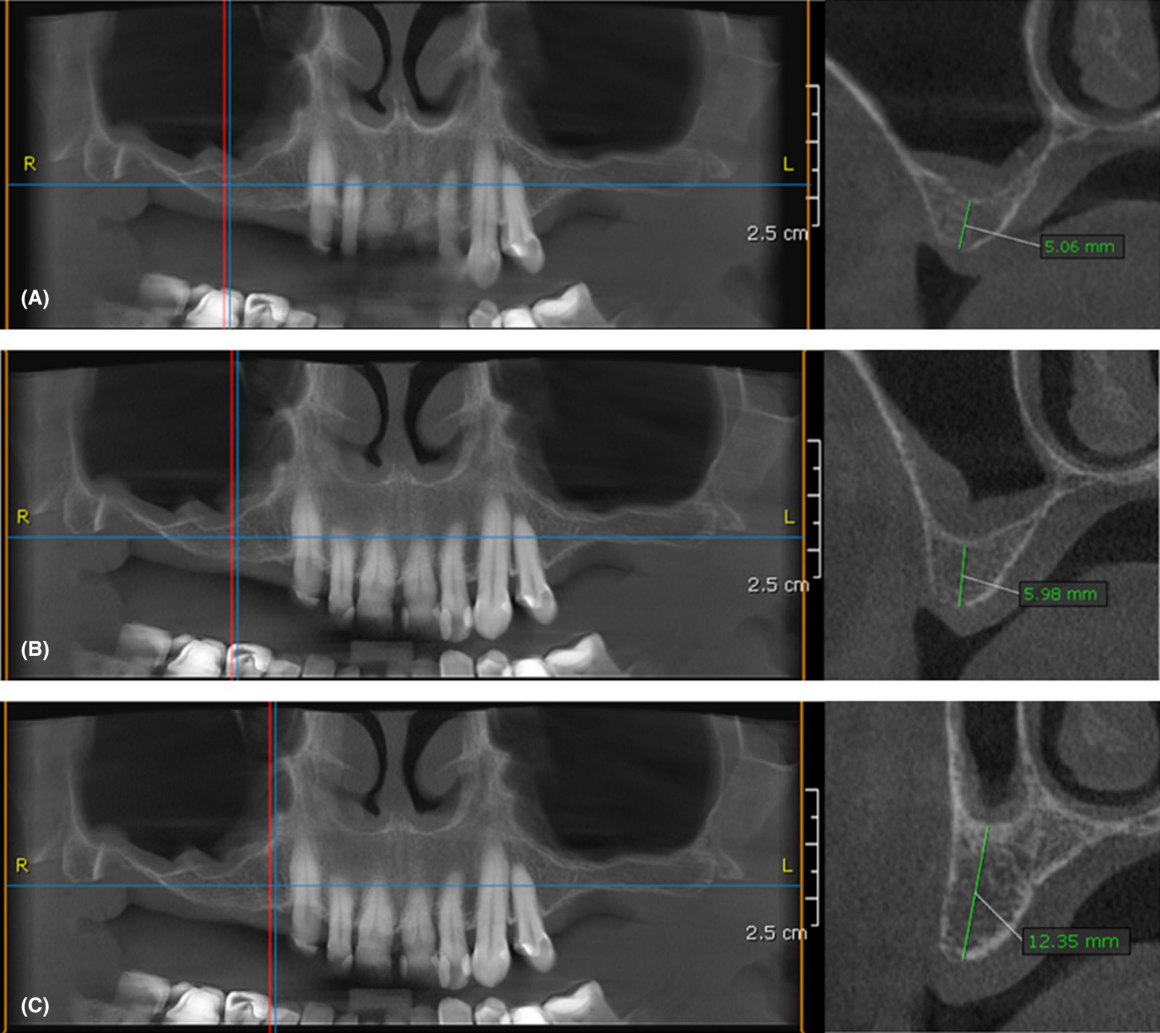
CBCT scan of the maxilla before implant placement. (A) Bone dimensions at the edentulous site of tooth no. 16. (B) Bone dimensions at the edentulous site of tooth no. 15. (C) Bone dimensions at the edentulous site of tooth no. 14.
